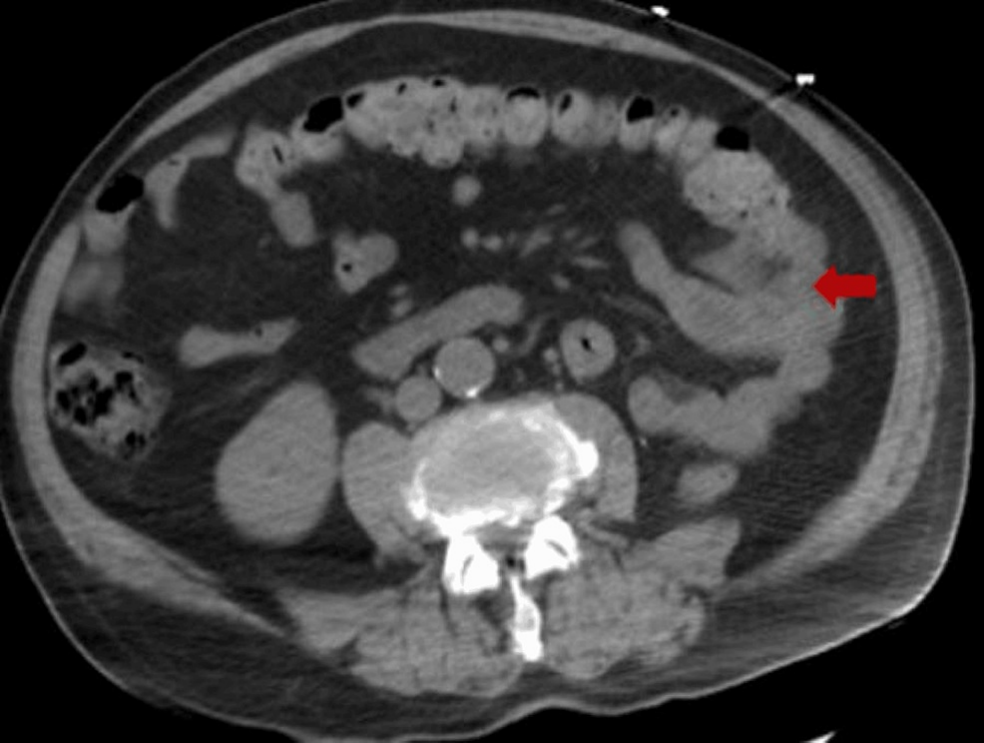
CT of the abdomen demonstrating colonic mass at the junction of the splenic flexure and proximal descending colon. CT, computed tomography
